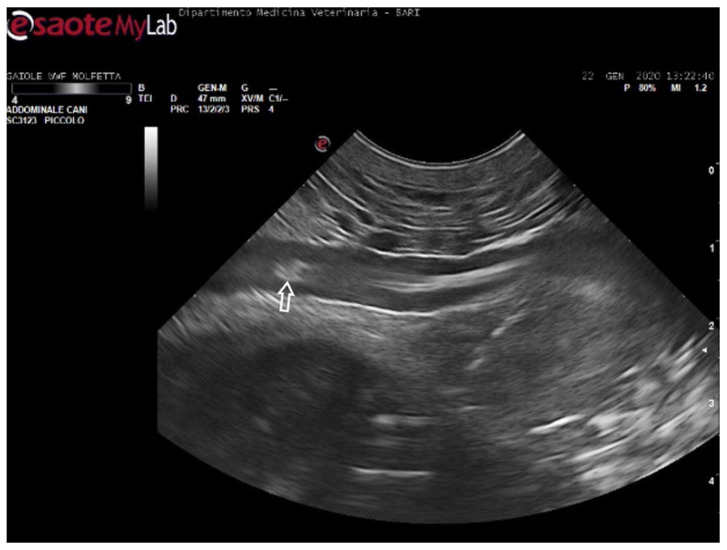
Ultrasound scan from the ventral cervical window revealing an aggregate of sizable microemboli (empty white arrow) in transit within the bloodstream of the left subclavian artery.
